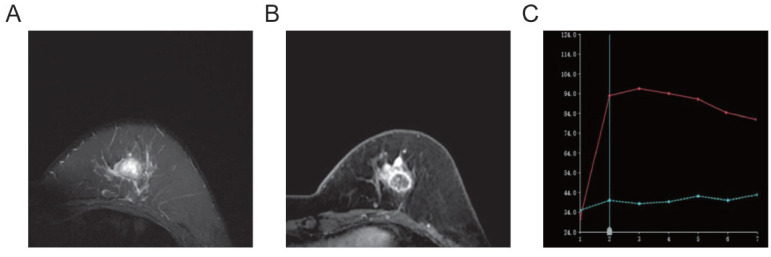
Left breast ductal carcinoma *in situ* (triple-negative subtype). The lesion shows high signal intensity at the center and iso- to low signal intensity at the margins on T2-weighted imaging (A). Enhancement of the lesion reveals a ring-like pattern (B). The TIC demonstrates an outflow-type enhancement (C).
